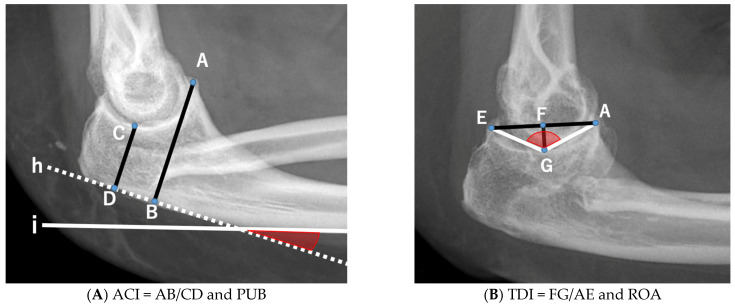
Index measurement of the elbow joint. A: tip of the coronoid process, AB: linear distance between point A and the posterior olecranon line, CD: minimal proximal ulna trochlear height, E: tip of the olecranon process, F: midpoint of the segment from AE, G: deepest point of the trochlear notch (determined by the intersection of a line perpendicular to AE and passing through point G and the trochlear notch), h: posterior olecranon cortex line, i: posterior ulna cortex line. (A) Proximal Ulnar Bow (PUB) is determined by lines h and i. (B) Radiographic Opening Angle (ROA) is determined by lines AG and GE. ACI, Anterior Coverage Index; TDI, Trochlear Depth Index.
